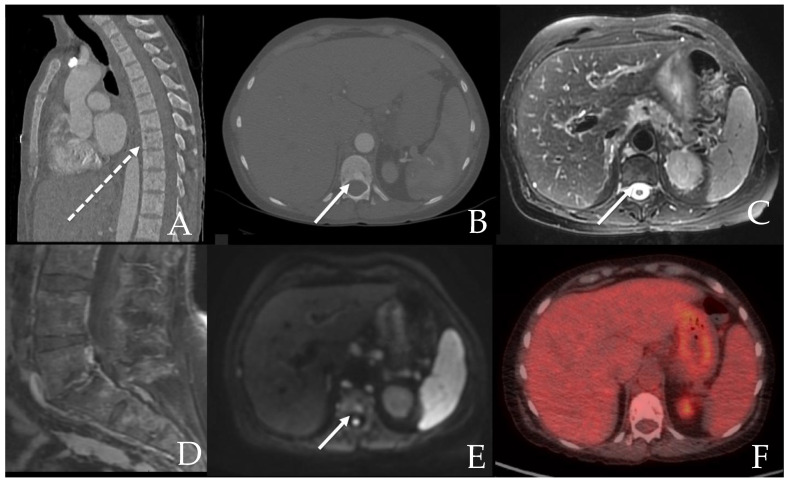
71 year old female with aggressive mastocytosis. Multimodality imaging demonstrates patchy sclerosis throughout the osseous structures on sagittal CT of the spine (A) with mild variable enhancement on post-contrast T1 weighted sagittal MRI of the spine (D). An example sclerotic lesion (arrow) on CT (B) is seen with mild T2 and DWI hyperintensity without PET hypermetabolism (C,E,F). A few lytic osseous foci (dashed arrow) were also noted (A).
